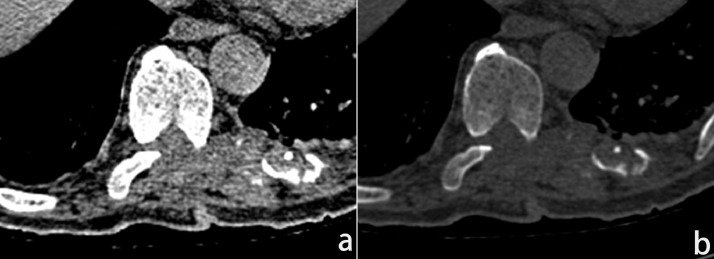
(a) CT soft tissue window. (b) CT bone window.
